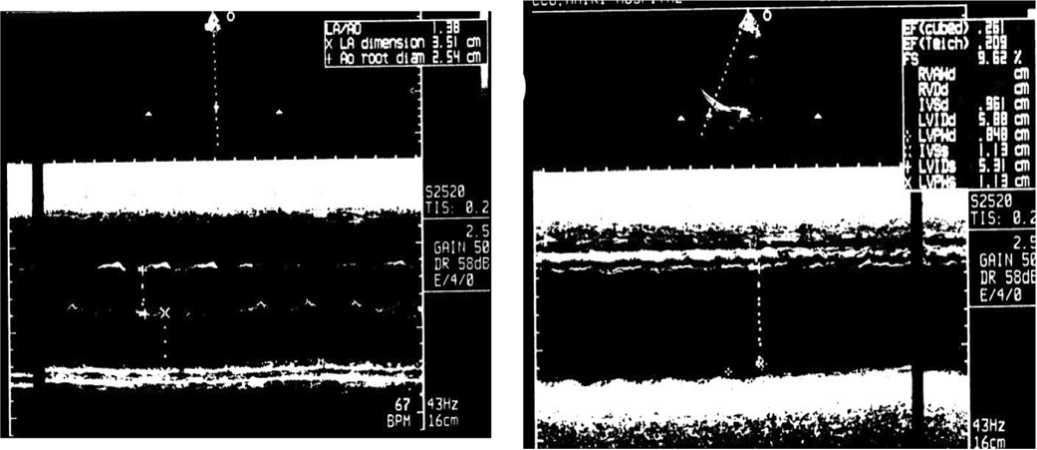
A transthoracic echocardiography showed a mildly dilated left ventricular with severe global left ventricular systolic dysfunction with an estimated left ventricular ejection fraction of 20 to 25%.
